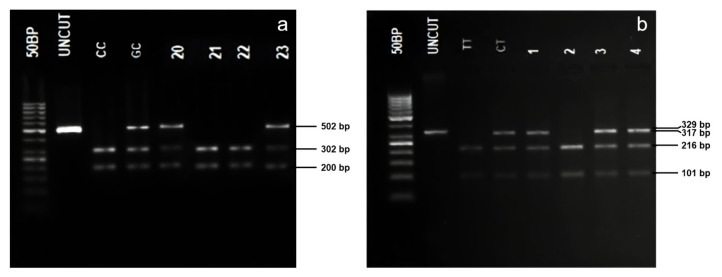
Analyses of the rs744166 (A) and rs4796793 (B) SNPs by PCR-RFLP method. a.) 50 bp is the molecular marker, UNCUT is the undigested PCR product (502 bp), CC genotype (302, 200 bp), GC genotype (502, 302, 200 bp), and 21–23 are patient samples; b.) 50 bp is the molecular marker, UNCUT is the undigested PCR product (329 bp), TT genotype (216,101, 8, and 4 bp), CT (317,216,101, 8, and 4 bp), and 1–4 are patient samples.
